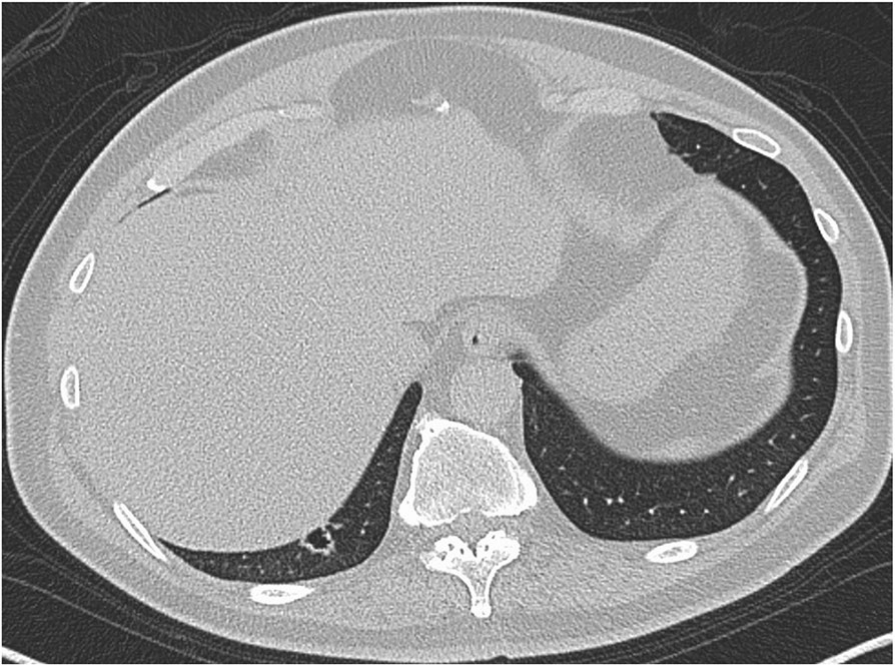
Chest computed tomography (CT) showing a cavitary lesion in S10b of the right lower lobe
